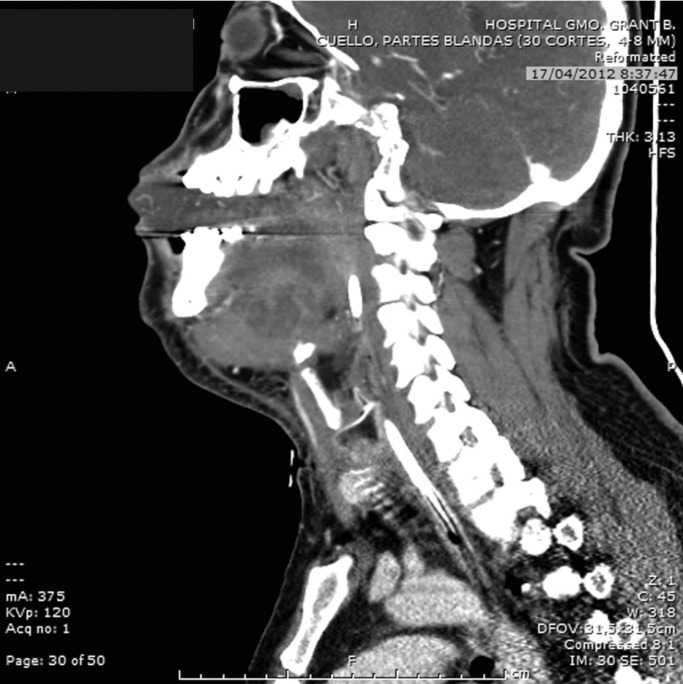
Computed tomography. Sagittal section showing upper airway elevation and protrusion of the tongue showing airway impairment and a large hypodense collection suggestive of a diffuse Ludwig’s angina infectious process.
